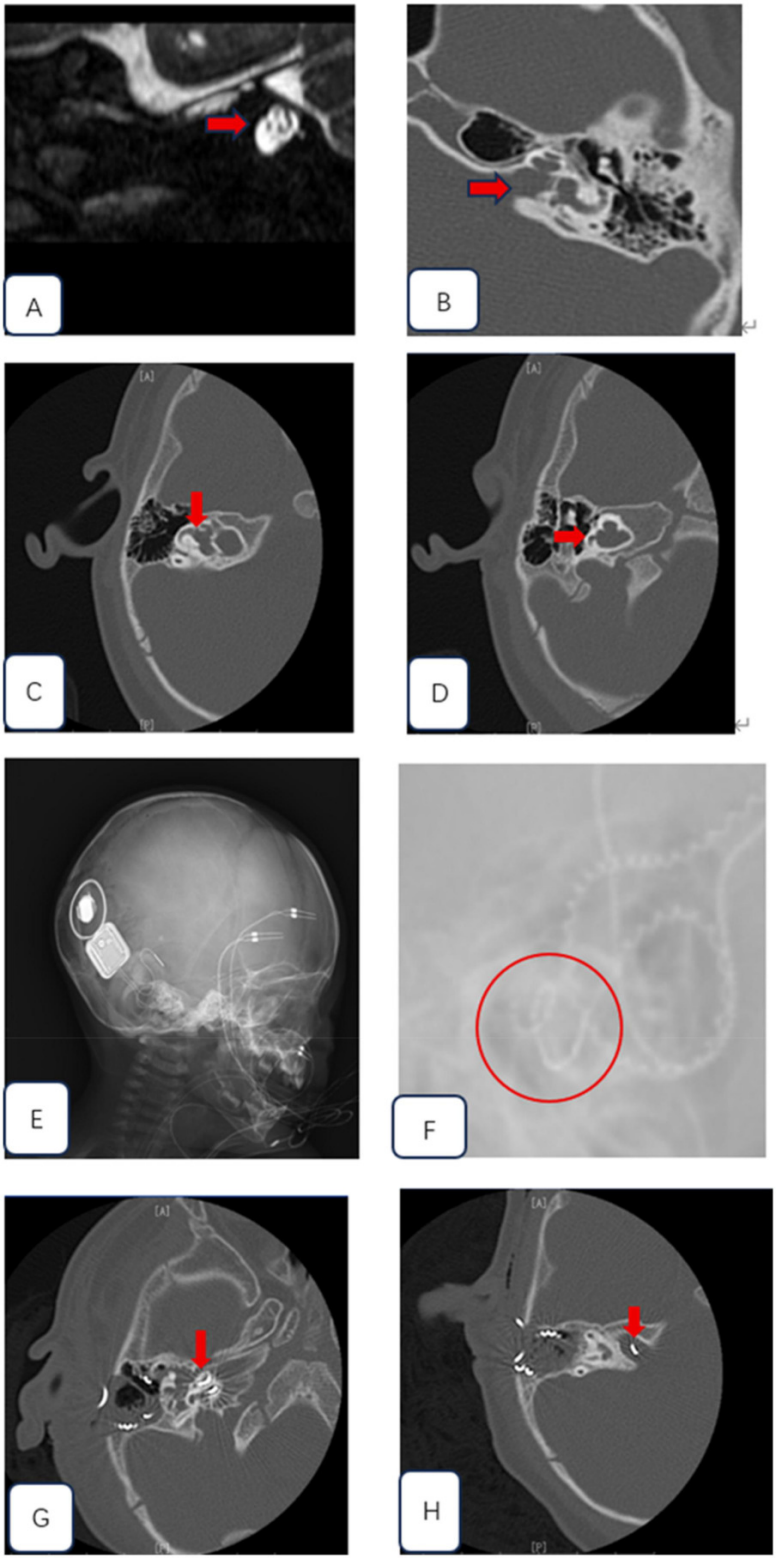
(A) axial, T2-weighted MR imaging of the internal auditory canal (ICA) suggest that all children had normally developed auditory nerves (B) widened internal auditory canal (IAC) (C) dilation of the vestibule with a cystic appearance (D) the cochlea was generally normal, the bony cochlear partition was present, and the modiolus was absent (E) cochlear imaging was used to confirm the position of the cochlear electrodes (F) intraoperative x-ray showed optimal placement of the cochlear electrode (G) postoperative CT scan confirmed the optimal position of the cochlear electrode H postoperative CT scan suggests misplacement of the cochlear implant electrode into the internal auditory canal.
